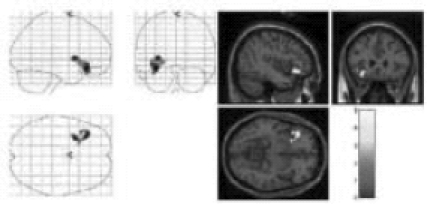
Left Inferior Frontal Gyrus Activation in the control subject without any telepathic ability [Mr. JS], while performing an unsuccessful telepathic task. On the left hand side, the activation is superimposed on a glass brain and on the right hand side, the activation [yellow] is superimposed on a structural MR image
